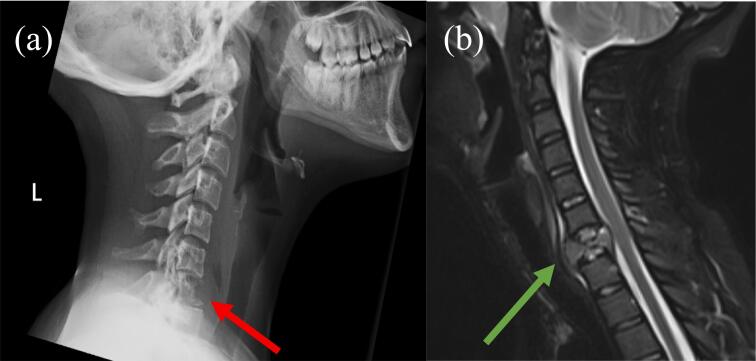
Preoperative radiographic assessment of the lesion. (a) Lateral X-ray of the cervical spine showing bone destruction at the anterior aspect of the vertebral body of C7. (b) An MRI showing a destructive lesion with vertebral plana involving C7 with associated mild cord comression.
